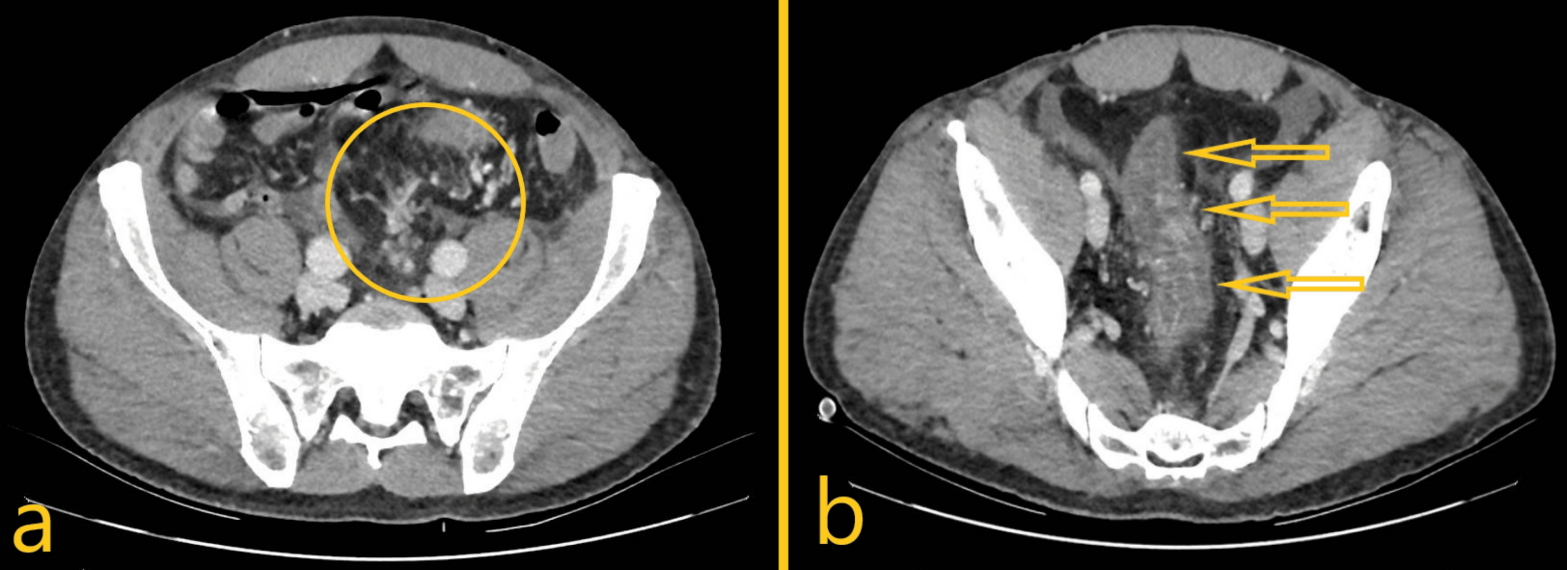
Abdominal computed tomography axial plane images. a) Early opacification of venous branches adjacent to the rectum and sigmoid, suggesting angiodysplasia (yellow circle) and maintained signs of non-thrombotic inferior mesenteric vein obstruction, findings that may be suggestive of other causes of bowel ischemia or inflammatory disease, such as IMHMV or enterocolic lymphocytic phlebitis; b) Persistence of thickening of the rectum and sigmoid walls (yellow arrows) with slight blurring of the surrounding fat, which may represent an inflammatory process. IMHMV: Idiopathic myointimal hyperplasia of the mesenteric veins
